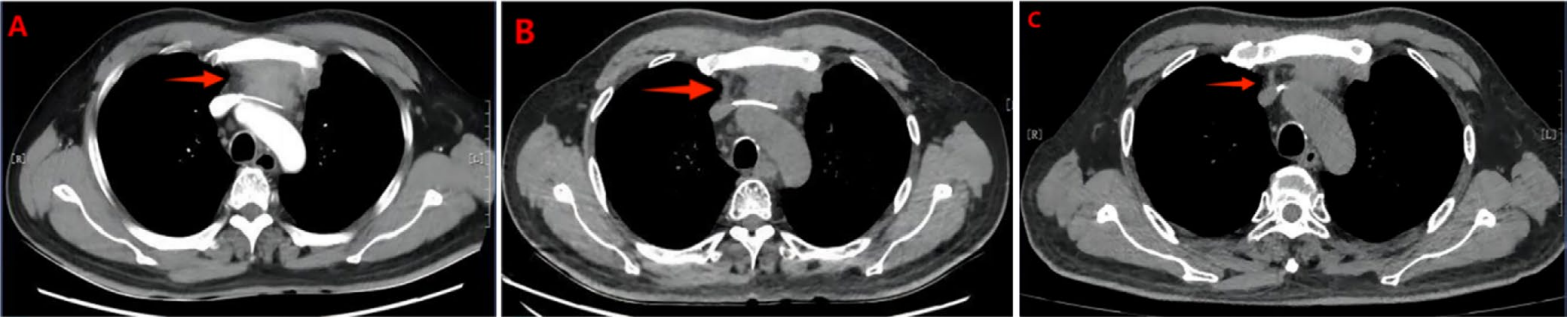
The dynamic changes of malignant lesions size as revealed by repeated chest CT scans before and after treatment. (A) Malignant lesions size before treatment. (B) Tumor lesion size after receiving 3 cycles of the EP regimen plus anlotinib. (C) Malignant lesions size after receiving camrelizumab.
